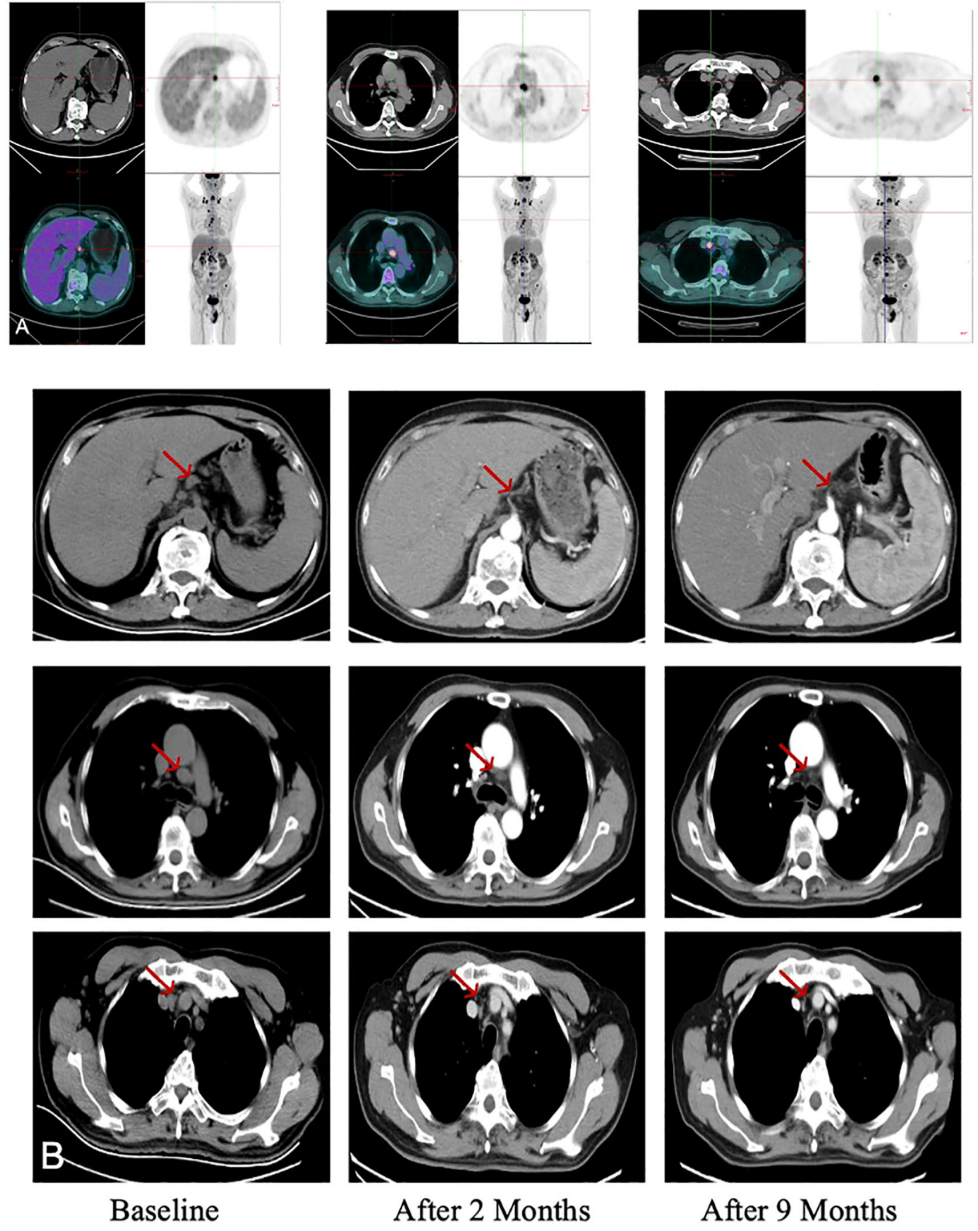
Comparative imaging demonstrates the evolution of metastatic lymph nodes. (A) Initial 18F-FDG PET-CT reveals extensive hypermetabolic lymphadenopathy in the mediastinal, gastrohepatic, and porta hepatis regions. (B) Computed tomography (CT) scans at baseline (pre-treatment) and at two and nine months post-treatment show serial regression of lymph node lesions. Red arrows indicate the regressing lesions at the specified sites.
